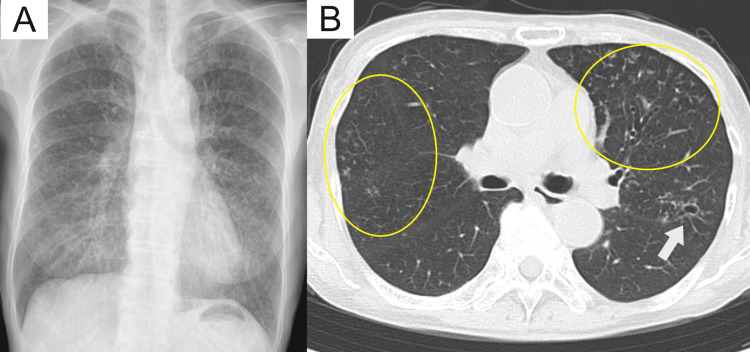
Chest radiograph and computed tomography after six months of treatment A: Chest radiograph shows improvement in diffuse nodular opacities; B: Chest computed tomography shows improvement in cavitary lesion (white arrows) and centrilobular nodules (yellow circles).
